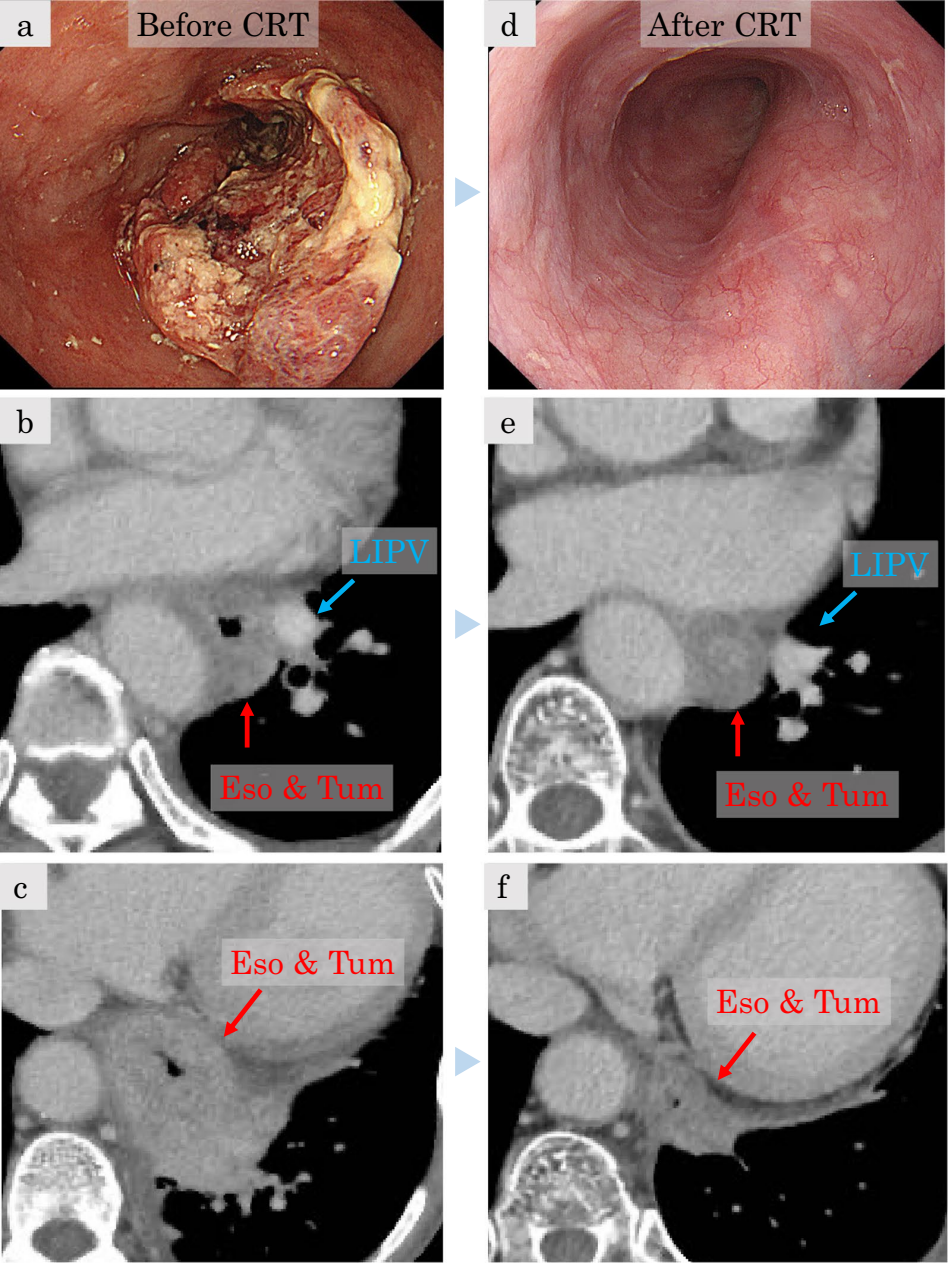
Initial upper endoscopy showing a circumferential type 2 tumor in the lower third of the thoracic esophagus. b, c Initial contrast-enhanced computed tomography (CT) revealing the tumor extending to the left lower lobe with a suspected invasion of the main trunk of the left inferior pulmonary vein (LIPV) (b). Eso & Tum, esophagus and tumor. d After induction chemoradiotherapy (CRT), upper endoscopy revealing only the tumor scar with stenosis. e, f After induction chemoradiotherapy, CT revealing shrinkage of the tumor without any lesion around the main trunk of the LIPV (e)
